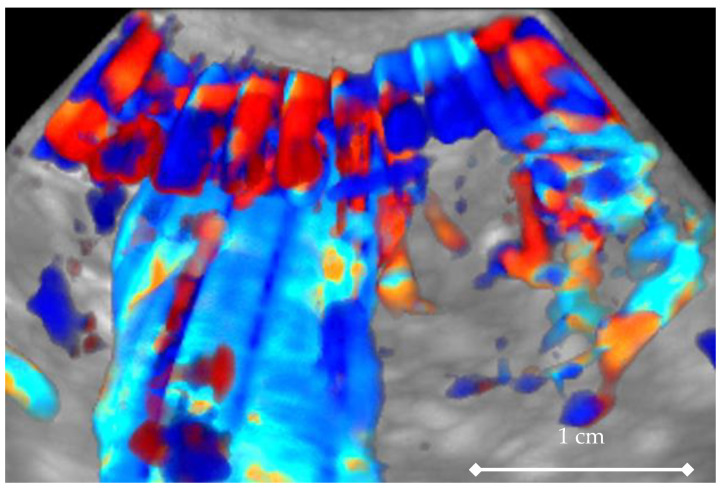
Color Doppler 3D magnified image of the transverse vessel (upper part of the image). The influence of periodically variable (systolic/diastolic) blood flow is visible as linear vertical irregularities in color. Creating a single record with ultrasound beam moving from left to right of the screen takes about 10s which covers about 10 heartbeat cycles.
